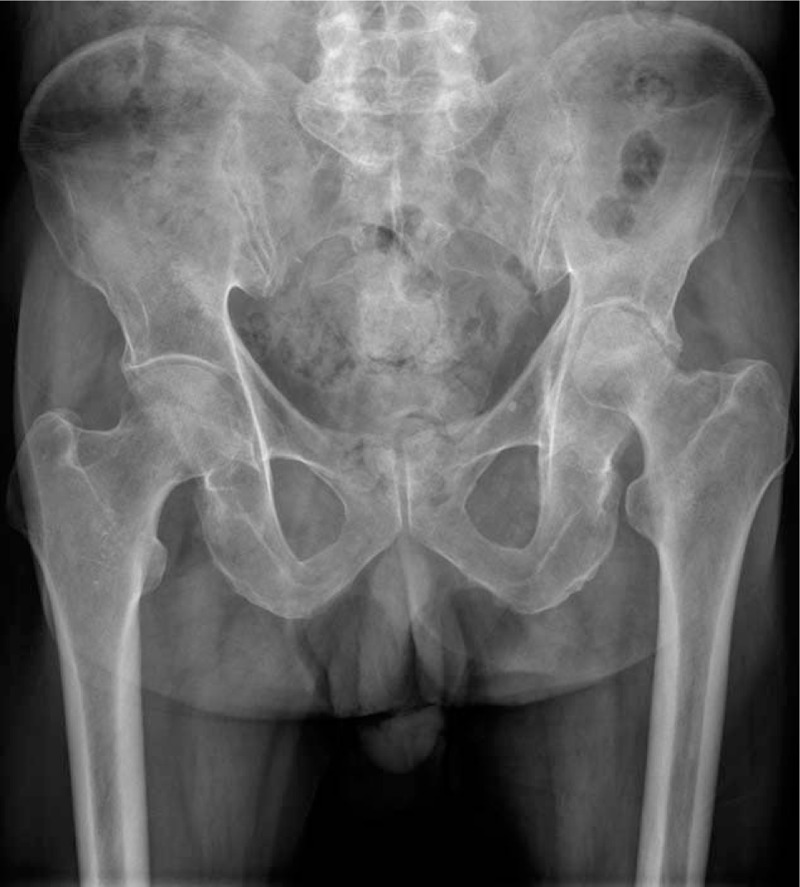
The preoperative X-ray of the pelvis. Preoperative X-ray of the pelvis. The left femoral head was dislocated and collapsed.
